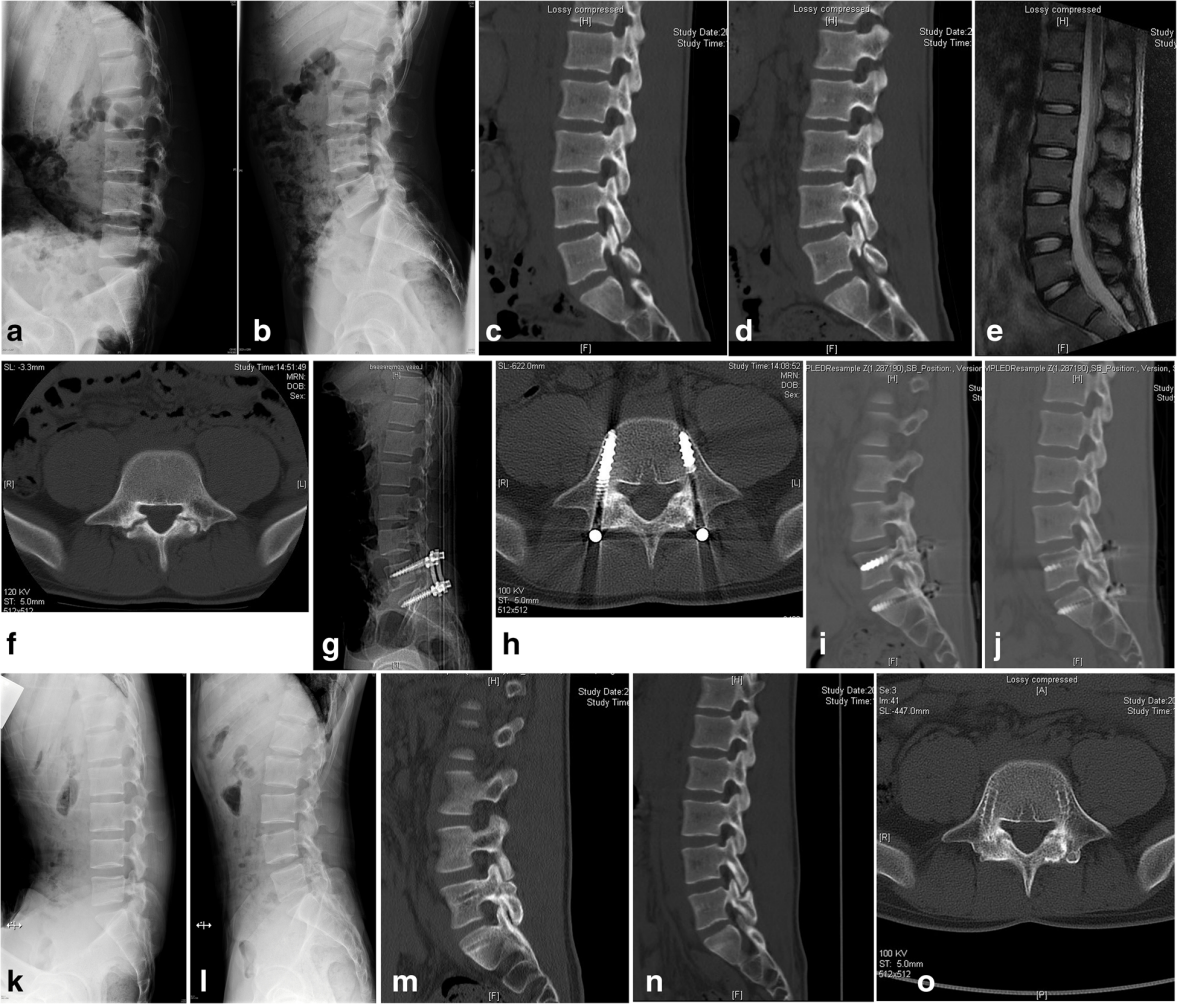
A young male patient of symptomatic bilateral spondylolysis repaired by autogenous iliac crest graft and temporary intersegmental pedicle screw fixation. a–c Preoperative CT scan showed L5 bilateral pars defect (CT classification Type II). d The signals of lumbar intervertebral discs were normal in T2-weighted sagittal MRI. e, f The ROM of L5S1 segment was 16.2° in dynamic lateral X-ray. g Lateral X-ray after surgery. h–j Bony continuity at the pars defect in axial and sagittal CT scan 12 months postoperatively. k, l There was 12.9° ROM of L5S1 segment with 79.6% motion preservation at 1 year after surgery. m–o Solid bone union showed in CT scan after fixation removal
